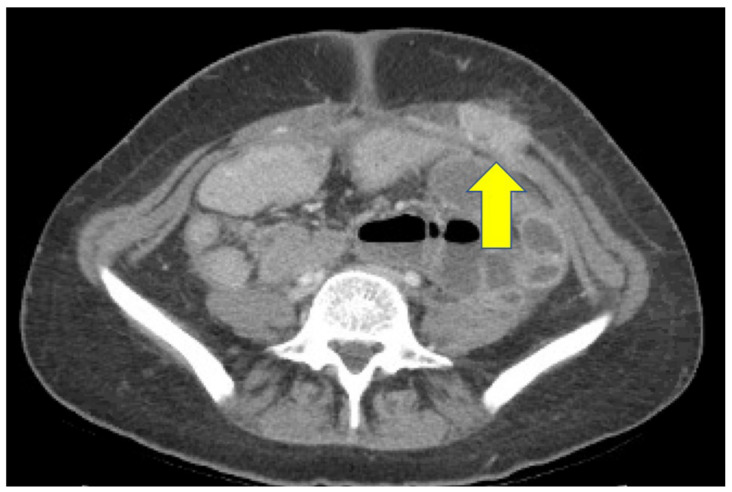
Axial CT image of case report patient demonstrated disease recurrence in left abdominal wall (yellow arrow).
